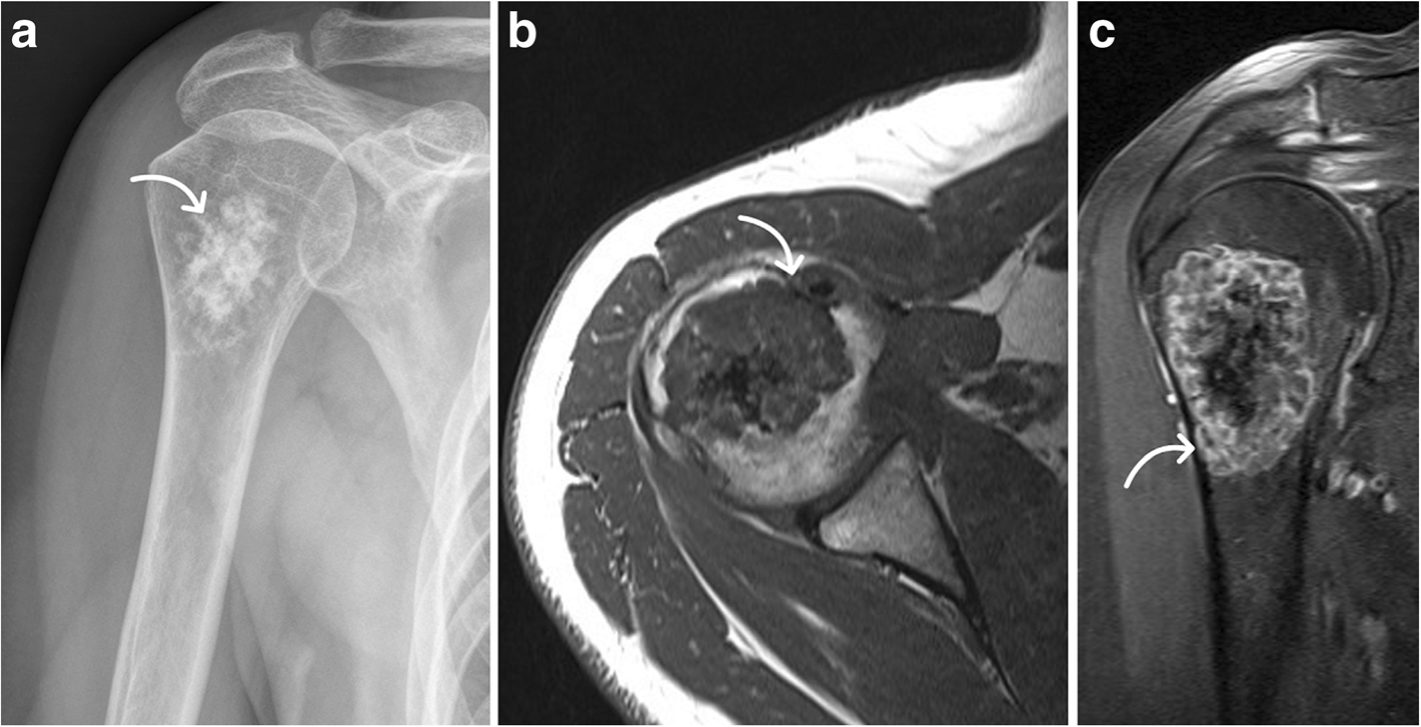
Painful large chondrogenic tumor at the proximal humerus prior to surgery with additional osteosynthesis. Plain radiograph (a) with typical popcorn-like calcifications (arrow) and T1-weighted axial MRI series (b) and T1-weighted contrast-enhanced coronal series (c) with endosteal scalloping (arrows) and reduced stability without significant differences compared to lesions treated without osteosynthesis (compare Fig. 1)
